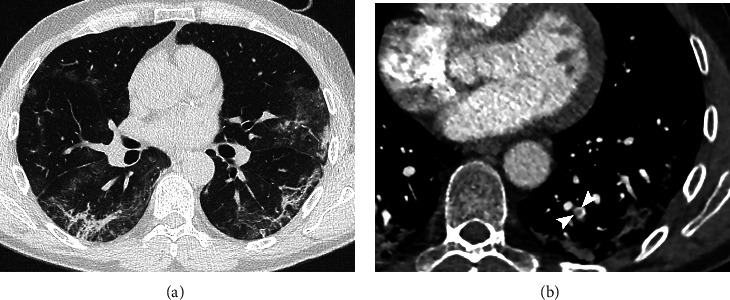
CTPA of a 58-year-old man with COVID-19 disease: (a) unenhanced HRCT shows multiple peripheral patchy GGO and bilateral linear opacities; (b) CTPA reveals an arterial filling defect in the subsegmental artery for left lower lobe (arrowheads).
